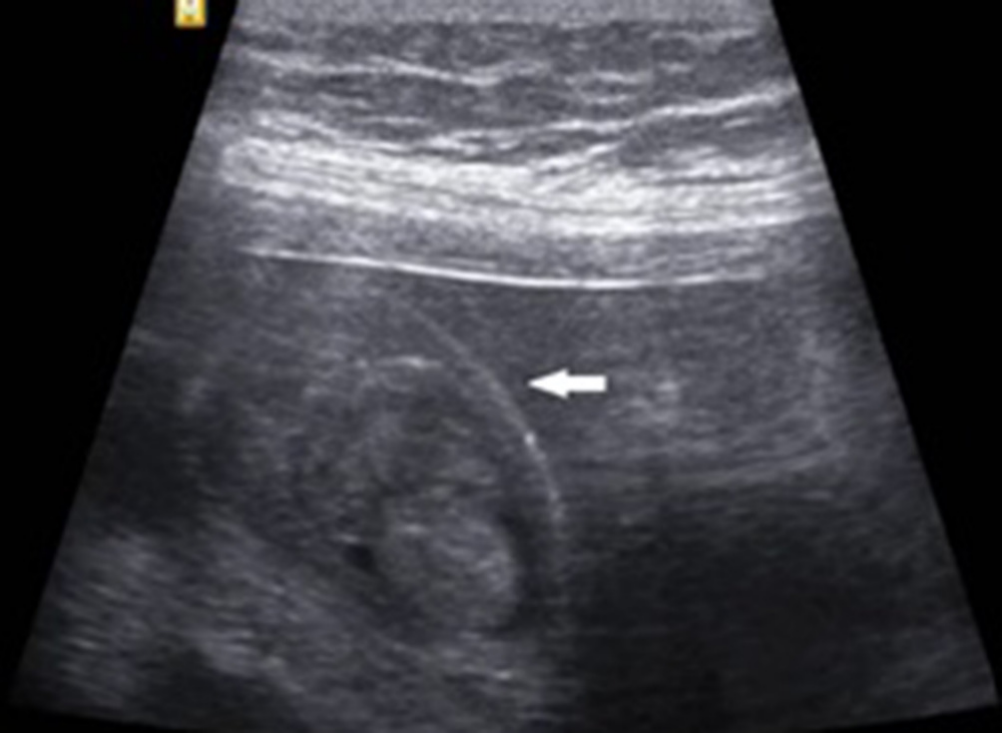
Longitudinal sonography of foetal scrotum showing an altered contour of the scrotum and movement of intrascrotal echoes. The surface of the scrotum is less convex (arrow) and concave (arrow in Figure 3) compared with Figure 1, where the surface is almost flat.
